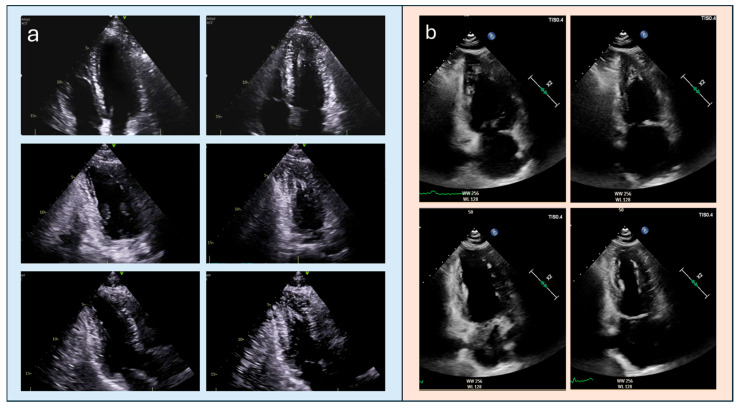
TTE assessment of long-axis views. (a): TTE long-axis views in a patient with ApHCM: correctly acquired apical four-, two-, and three-chamber views at end-diastole (right) and end-systole (left), demonstrating appropriate visualization of the true apex. (b) TTE long-axis views in a normal patient: example of apical foreshortening in the apical two-chamber view (upper row), which may falsely suggest apical hypertrophy; the correctly obtained view (lower row) restores the true apical geometry and demonstrates normal apical morphology. TTE, transthoracic echocardiography; ApHCM, apical hypertrophic cardiomyopathy.
